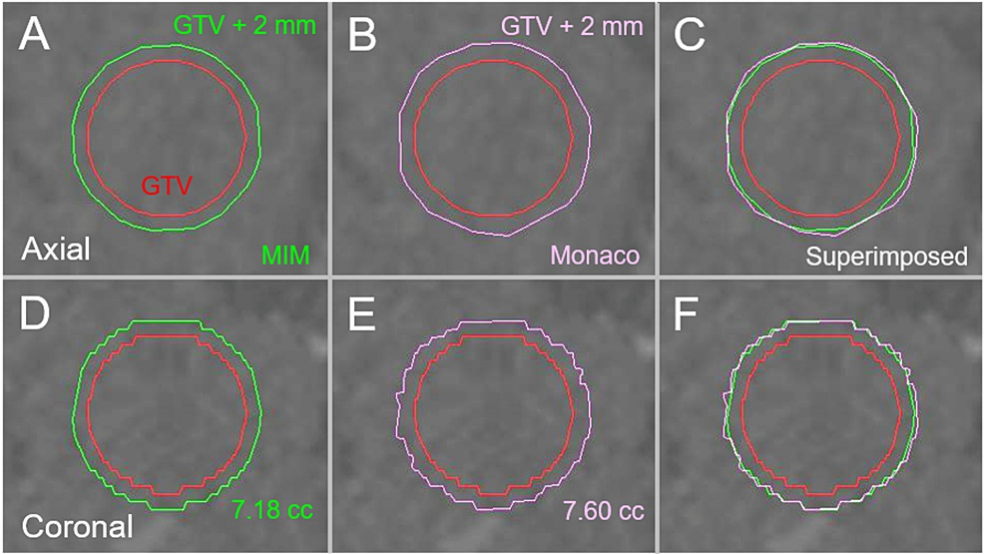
The differences in margin addition functions between two planning systems in a 20-mm sphere model. The images show the target contours superimposed onto non-contrast-enhanced computed tomography (CT) images (A-F); axial images (A-C); coronal images (D-F); by MIM Maestro (A, D); by Monaco (B, E); and the superimposed images (C, F). Isotropic 2-mm margins were added to the 20-mm sphere model by MIM Maestro and Monaco, respectively. The 2-mm margin unevenness is more noticeable in Monaco than in MIM Maestro. Additionally, the 2-mm margin-added volume by Monaco is slightly larger than that by MIM Maestro. GTV: gross tumor volume; GTV + 2 mm: GTV evenly expanded by 2 mm; MIM: MIM Maestro.
